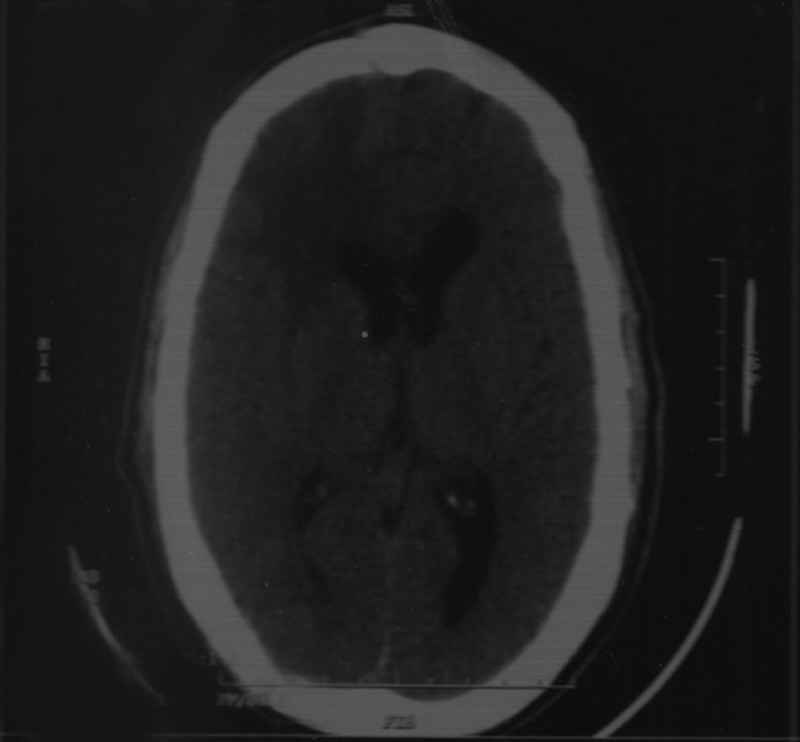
Postoperative noncontrast computed tomography (CT) scan, showing complete excision of the tumor.
